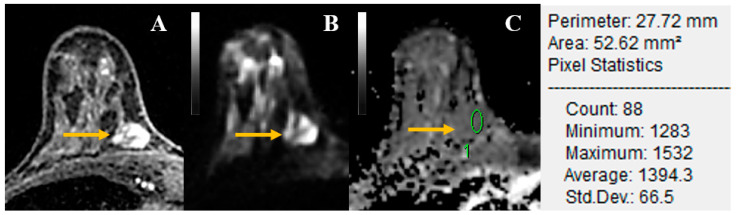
Axial MR images of a 48-year-old woman with a 14-mm benign mass in the right breast, of which biopsy yielded fibro-adenomatoid changes (yellow arrows). (A) Axial dynamic contrast-enhanced image depicts a heterogeneous, oval, and circumscribed enhancing mass in the right breast corresponding to a heterogeneous hyperintense lesion on axial diffusion-weighted imaging (DWI) at a b value of 800 s/mm^2^; (B). (C) Correlative parametric apparent diffusion coefficient (ADC) map with a region of interest (ROI) placed within the darkest part of the lesion and ROI information. ADC values are expressed in mm^2^/s. This lesion was heterogeneous vs. non-enhancing septa and therefore characterized as BI-RADS 3 and 3 based on the DWI score in the consensus reading of radiologists.
